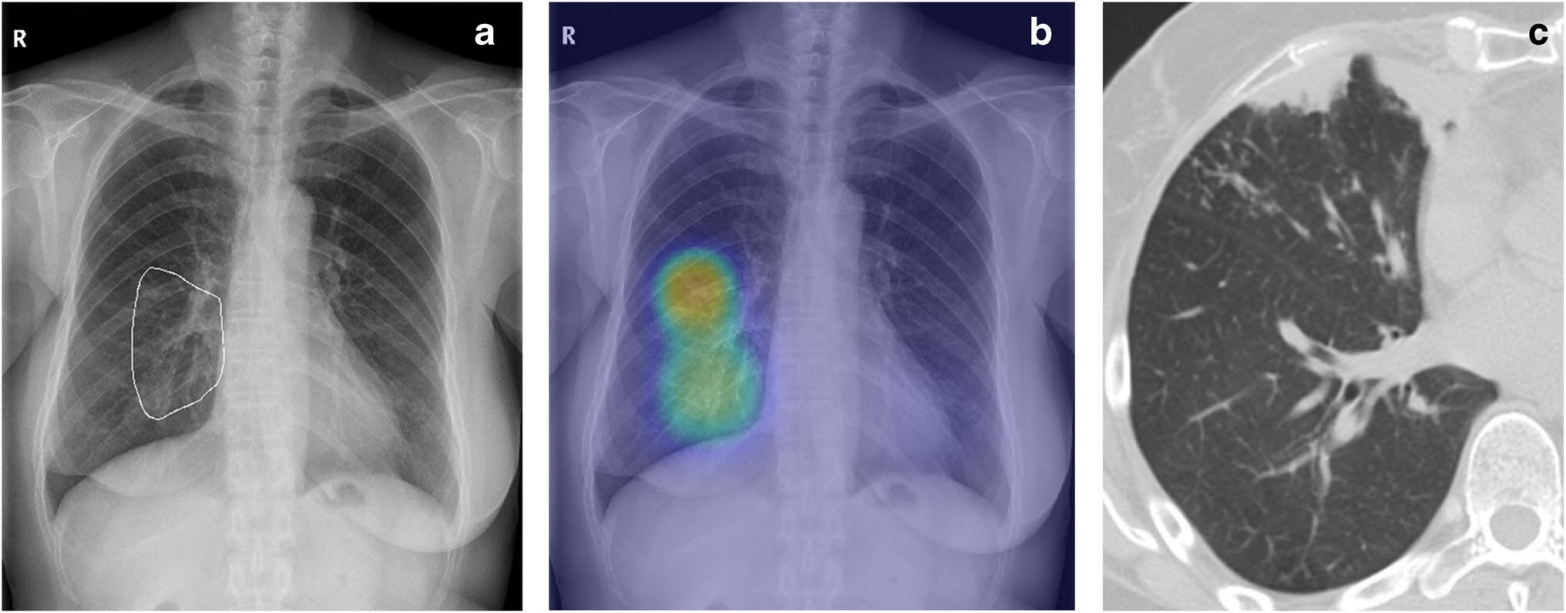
A 54-year-old woman with pneumonia in the right lower lung zone. Chest radiography demonstrated ill-defined ground-glass opacity or consolidation in the right para-hilar area, which was marked with a white outline as the reference standard. a The AI solution correctly detected the lesion with a probability value of 69%. b Chest CT without contrast enhancement shows consolidation and tiny ill-defined nodules in the right middle lobe. c Among the 12 observers, seven could detect the lesions without AI assistance. With the use of an AI solution, all observers could detect the lesions. The AI solution led to accurate detection of pneumonia on chest radiographs in the case of five observers (42%), including two pulmonologists, one thoracic radiologist, one general radiologist, and one radiology resident
